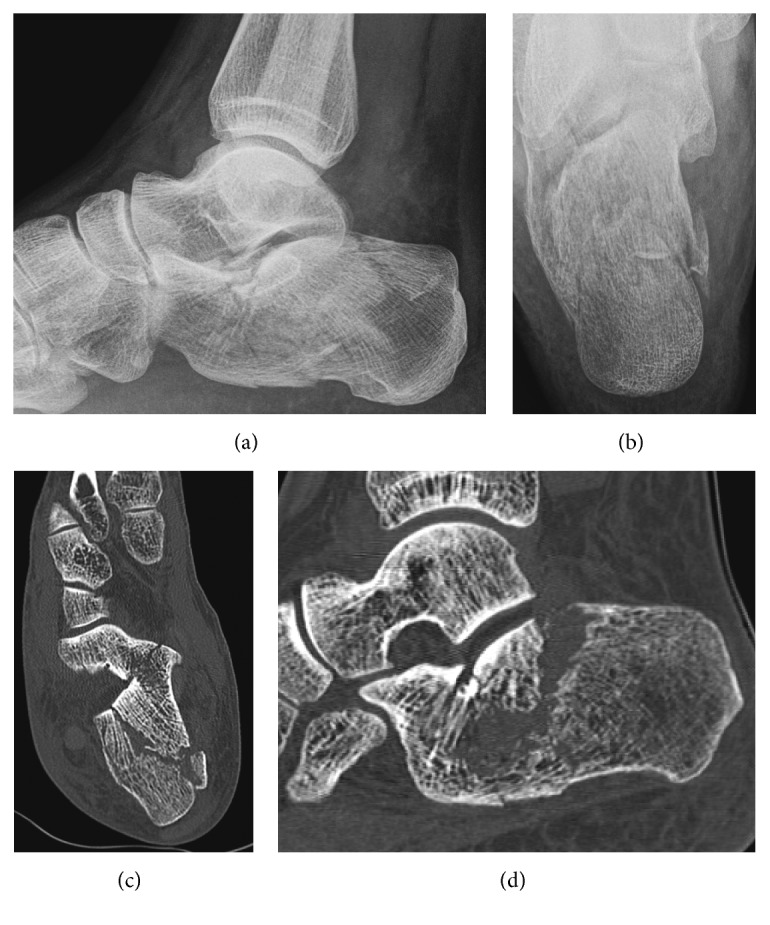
Preoperative imaging of the left foot. The upper part revealed a multifragmentary fracture of the calcaneus, with a Boehler's angle of 5 degrees. The CT scan permitted to classify the fracture as a Sanders type IIBC.
